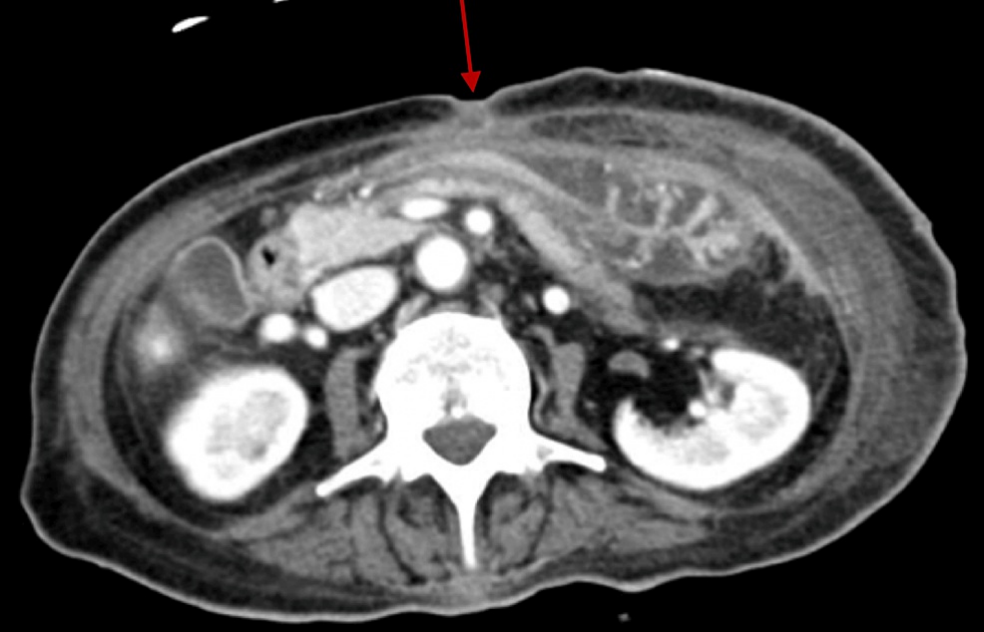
CT scan of the abdomen showing interval closure of the stoma (arrow).
